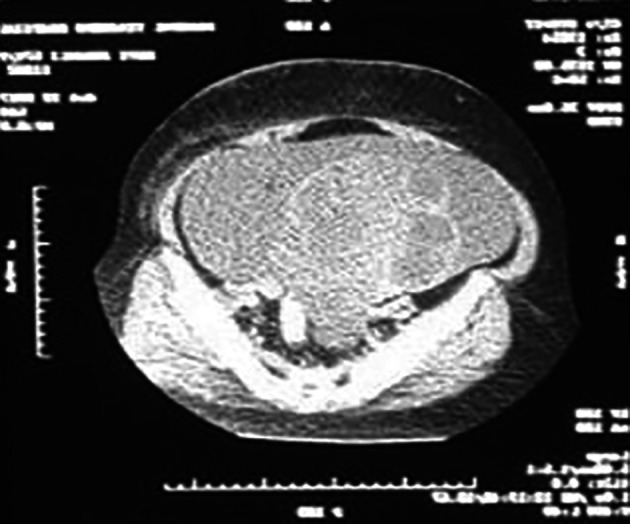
CT scan of the abdomen and pelvis showing the complex ovarian cysts.
